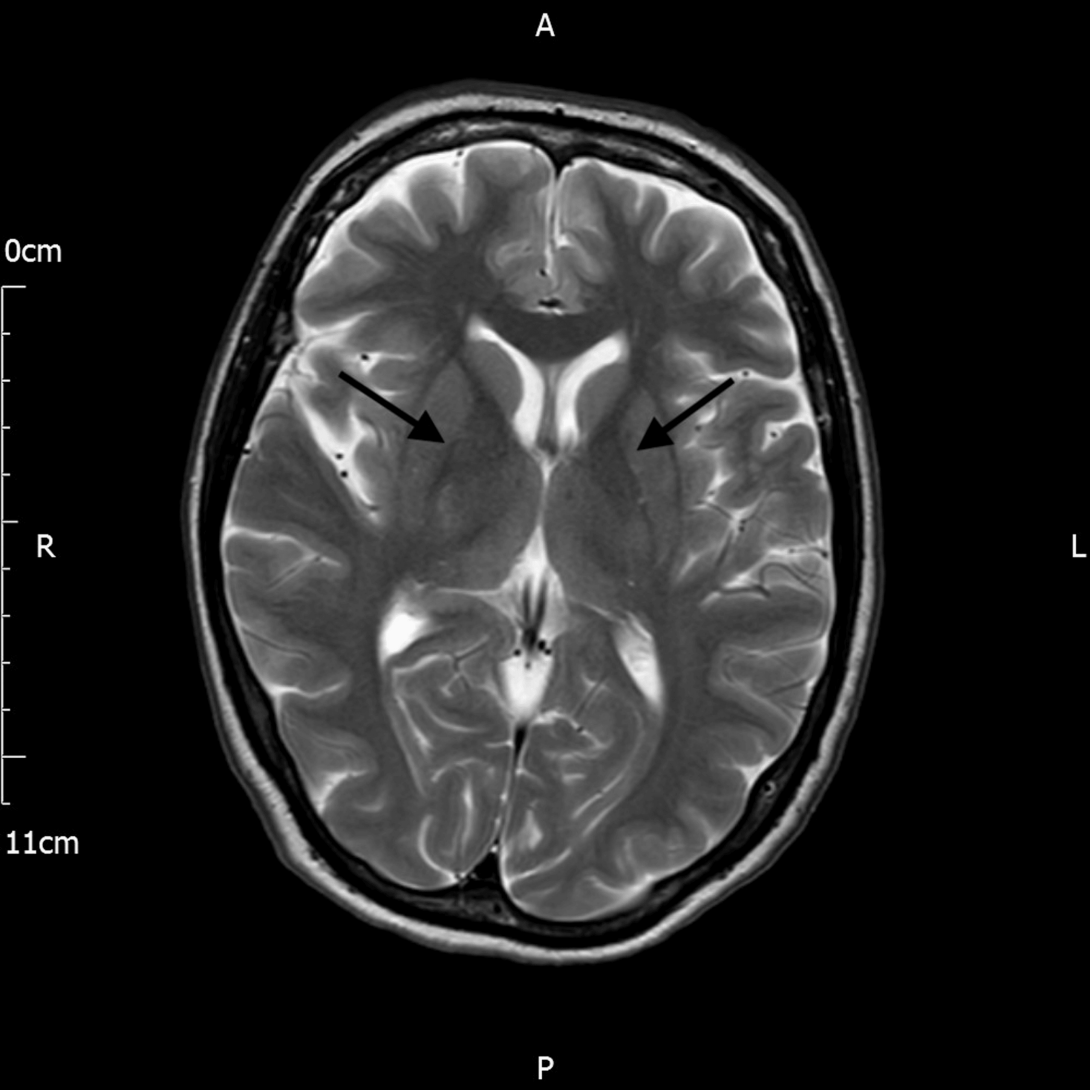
T2-weighted axial MRI of the brain The arrows indicate the absence of hyperintensity in the region of the basal ganglia
